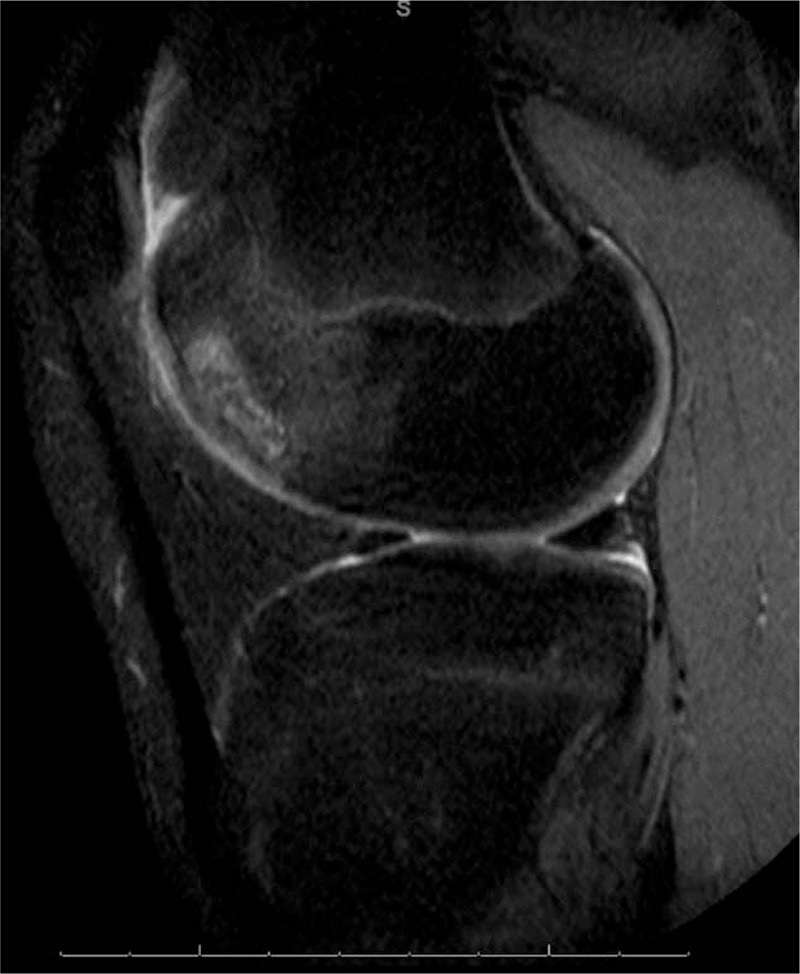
Sagittal magnetic resonance imaging demonstrating lateral trochlear osteochondritis dissecans lesion.
